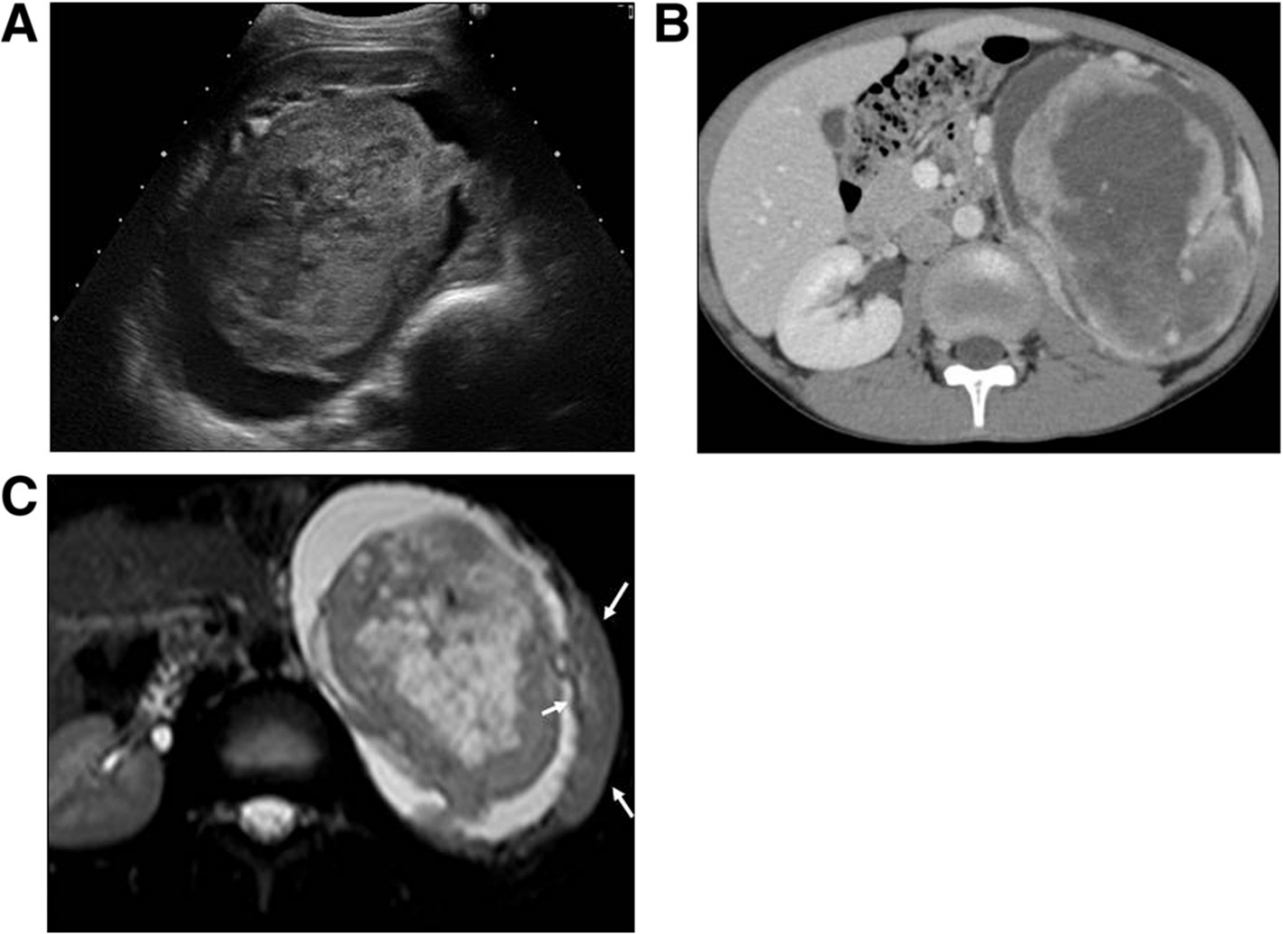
Images of the left kidney tumor. (A) Ultrasonography showing a solid tumor in the left kidney. The tumor borders were clear, and the tumor measured 11 × 10 × 8 cm. We detected mixed echogenicity inside the tumor. (B) Contrast-enhanced computed tomography showing a solid tumor with clear borders and contrasted disproportionate, with no lymph node swelling or tumor embolism. (C) Magnetic resonance imaging (T2-weighted) showing necrosis and hemorrhage inside the tumor. The tumor was suspected to have originated from left kidney tissue (arrow; normal kidney).
